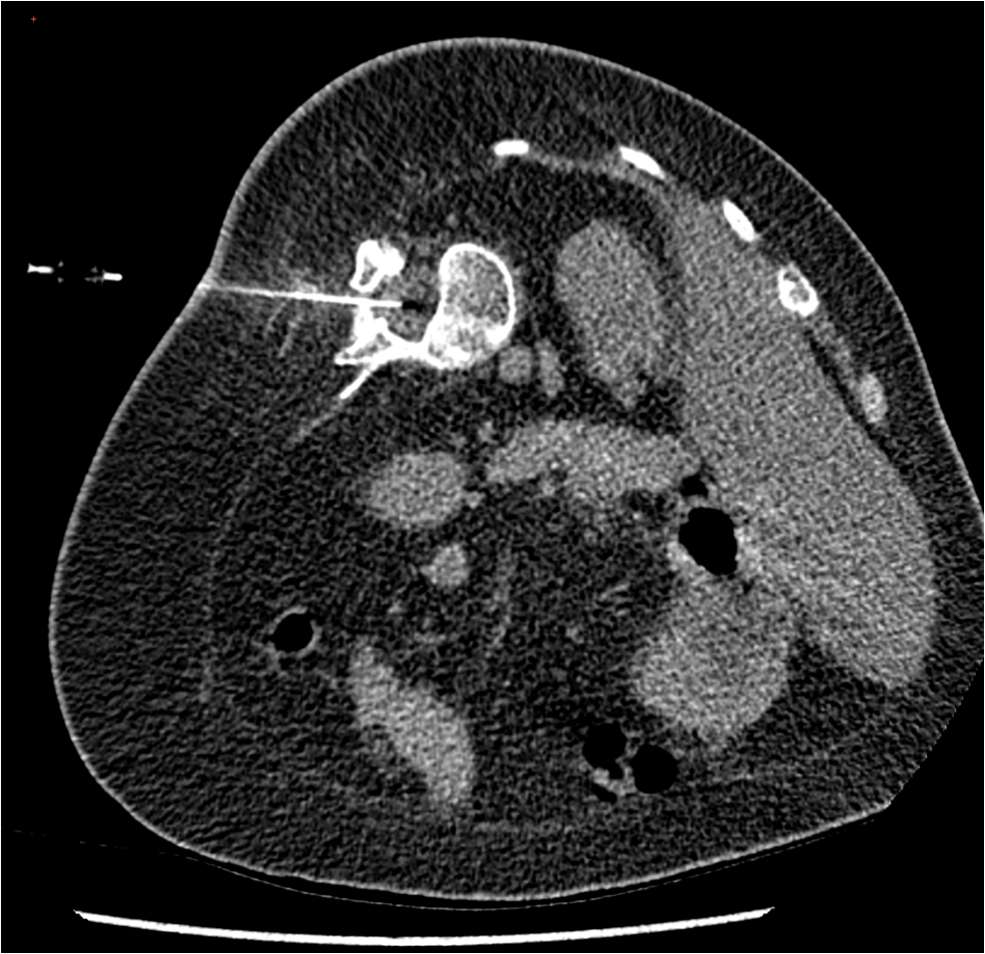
CT-guided intrathecal nusinersen injection—ultra-low-dose protocol
